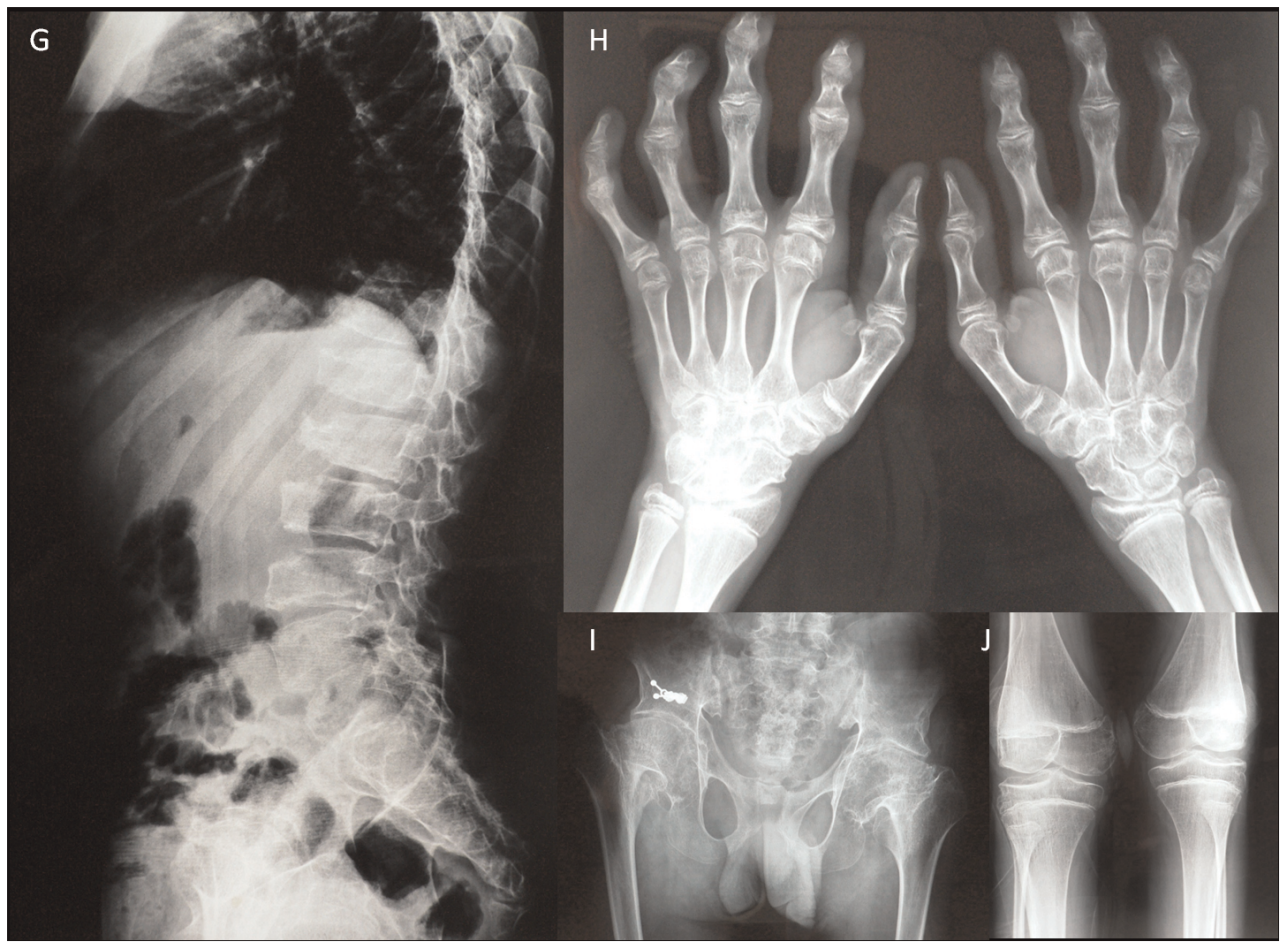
The X-ray images of patient II 1 in family 1. (G) Platyspondyly of the lumbar spine: the vertebral bodies are wedged anteriorly, and there are narrow disc spaces, irregular vertebral end-plates and short pedicles; (H) enlargement of the epiphyseal and metaphyseal portions of the metacarpals and phalanges; (I) bilateral femoral neck fractures and enlargement of the capital femoral epiphyses; (J) an enlargement of genual epiphyses.
